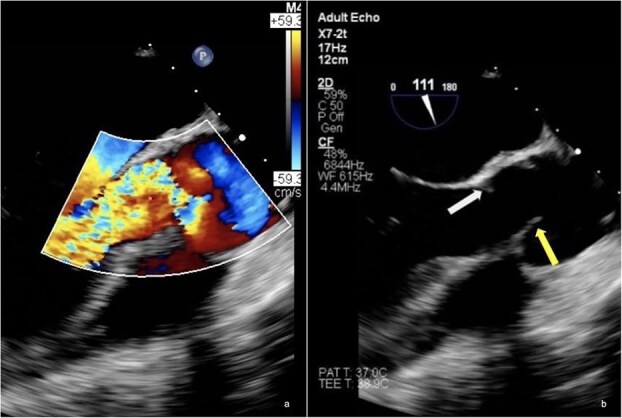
(A) TOE Doppler of aortic valve exhibiting severe degree of aortic regurgitation. (B) Mid-oesophageal aortic valve long axis view with doppler. Absence of coaptation between non-coronary and right-coronary cusps, indicating, most likely, a mechanical failure tear in the NCC/RCC commissural region. White arrow—Absent NCC, yellow arrow—Normal RCC.
